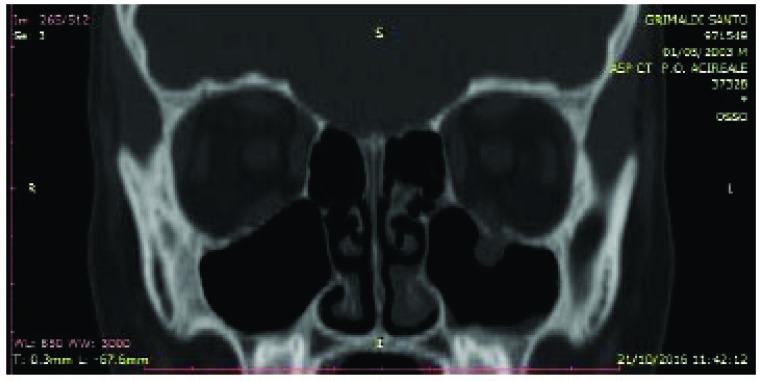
Preoperative CT coronal projection. The left orbital floor seems to be collapsed.
